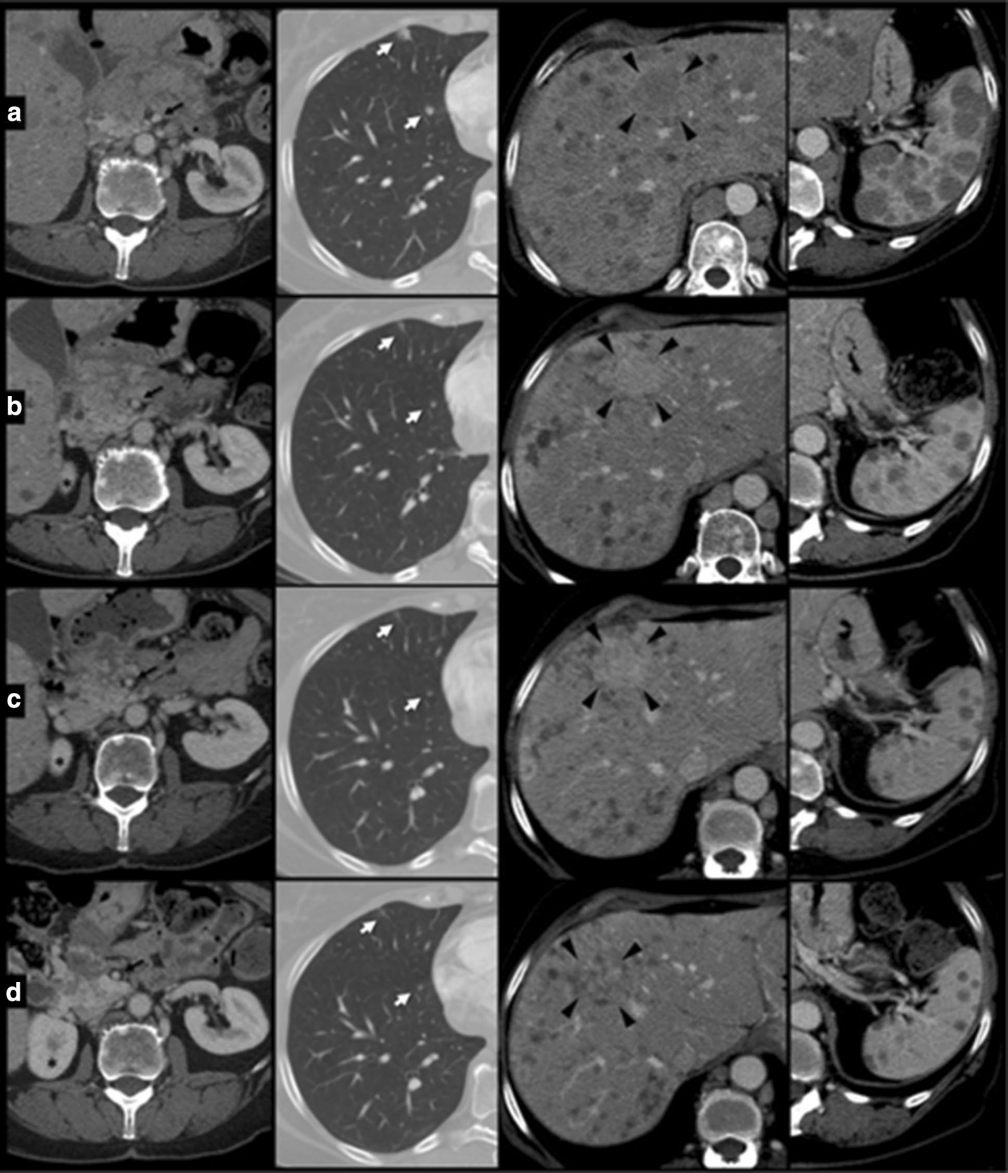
Changes of lesions over time in pancreatic head region, lung, liver and spleen at time 0 (a) and at 4 (b), 7 (c), 10 month (d) follow up examinations. Pancreas: enlargement of pancreatic head region was observed at time 0, with strongly inhomogeneous density, indistinct pancreatic margins and surrounding retroperitoneal fat stranding; some peripancreatic lymph nodes enlarged were detected. Following CT examinations show gradually decrease of pancreatic swelling with better definition of parenchymal lesions, going towards progressive regression e colliquation. Note progressive appearance of right kidney’s *upper pole asterisk*, according to reduction of displacement effect by liver. Furthermore, note the displacement of superior mesenteric artery (*black arrow*) to right side due to reduction of pancreatic swelling. Lung: progressive volume reduction of metastatic lesions (*white arrow*) in anterior basal segment of right lower pulmonary lobe. Liver: one of the major lesions of the liver at the IV segment (*arrowhead*) underwent progressive volume reducing ad enhancement pattern. Spleen: volume reduction of multiple metastatic lesions
